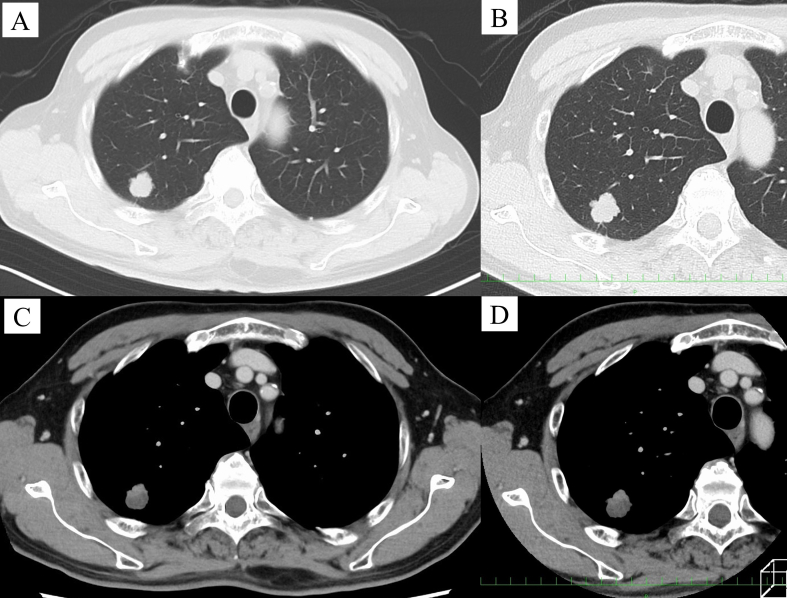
(A-D) Computed tomography images showing a well-defined, low-attenuation lobulated nodule in the right upper lobe.
